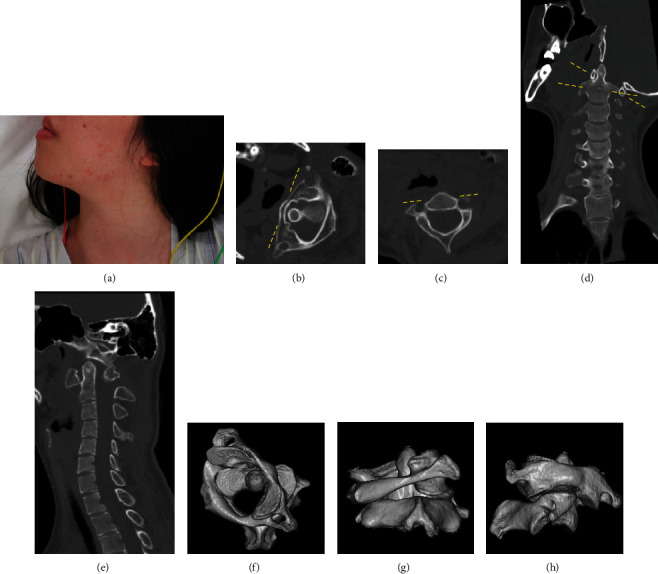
Patient position before reduction (a) and CT of the cervical spine on admission (b–h). (b) Axial view of C1, (c) axial view of C2, (d) coronal view, (e) sagittal view, and (f–h) three-dimensional reconstruction. The C1-C2 rotatory angle was 60 degrees.
